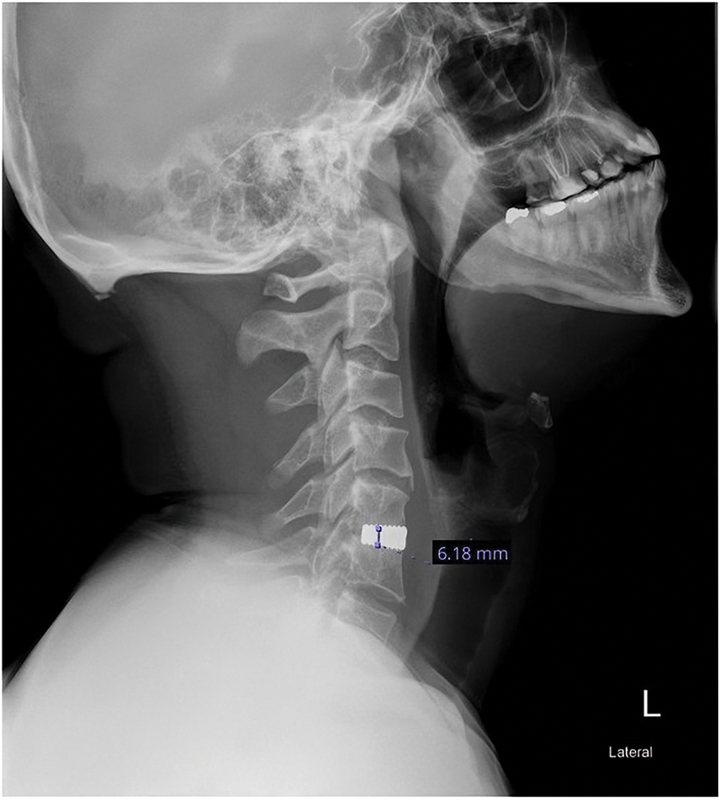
Lateral X-ray of the cervical spine with stand-alone cage, the blue line indicating the midpoint of the lower margin of the upper vertebra and the upper margin of the lower vertebra at the fusion site for which the cage subsidence is measured.
